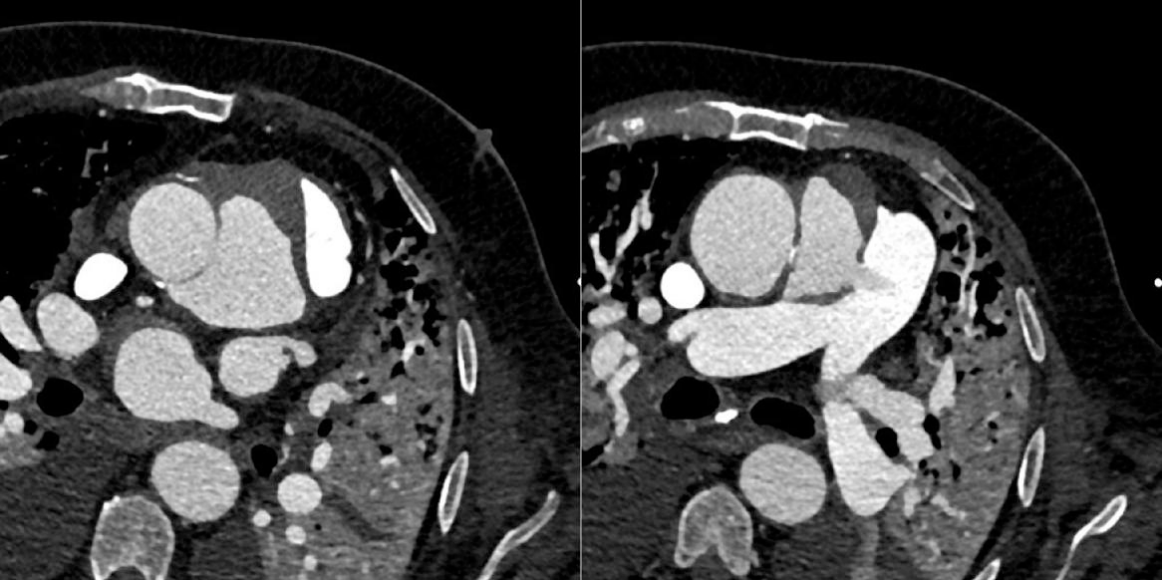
Contrast computed tomography angiography images in oblique axial reconstruction demonstrating the voluminous pseudoaneurysm of the aortic root (left) and the aortopulmonary fistula (right).
